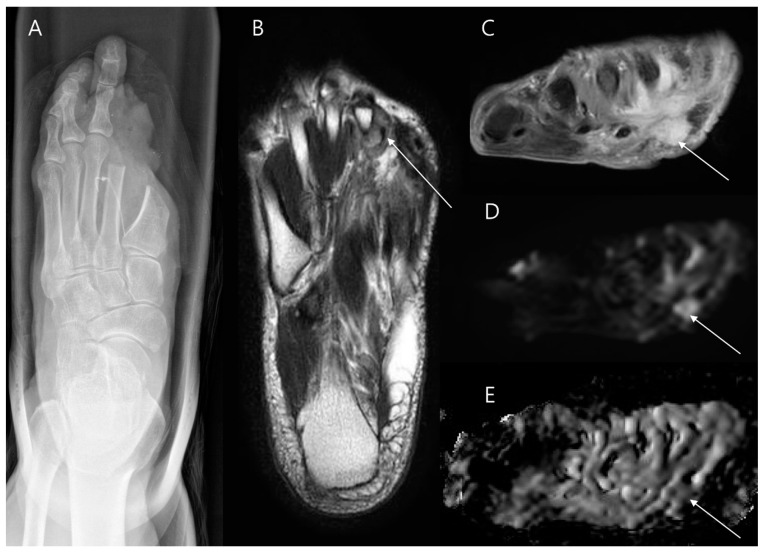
Recurrent mass in a patient with clear cell sarcoma after amputation with flap coverage. (A) Post-amputation state is shown. (B) Postoperative 3-month coronal T2-weighted MR image shows a nodular lesion near the flap (arrow). (C) Axial T1-weighted fat-suppressed enhanced MR image highlights a discrete enhancing nodule near the flap (arrow). (D,E) This lesion (arrows) demonstrates high signal intensity on the high b-value image of diffusion-weighted imaging and low signal intensity on the apparent diffusion coefficient map, indicating a recurrent mass.
